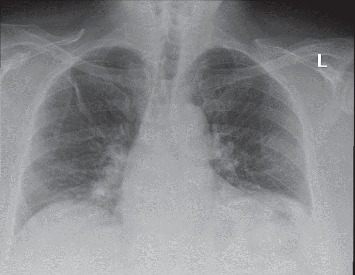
A chest X-ray with an anterior-posterior view: diagonal band-like density in the right upper lobe and linear densities at the bases.
